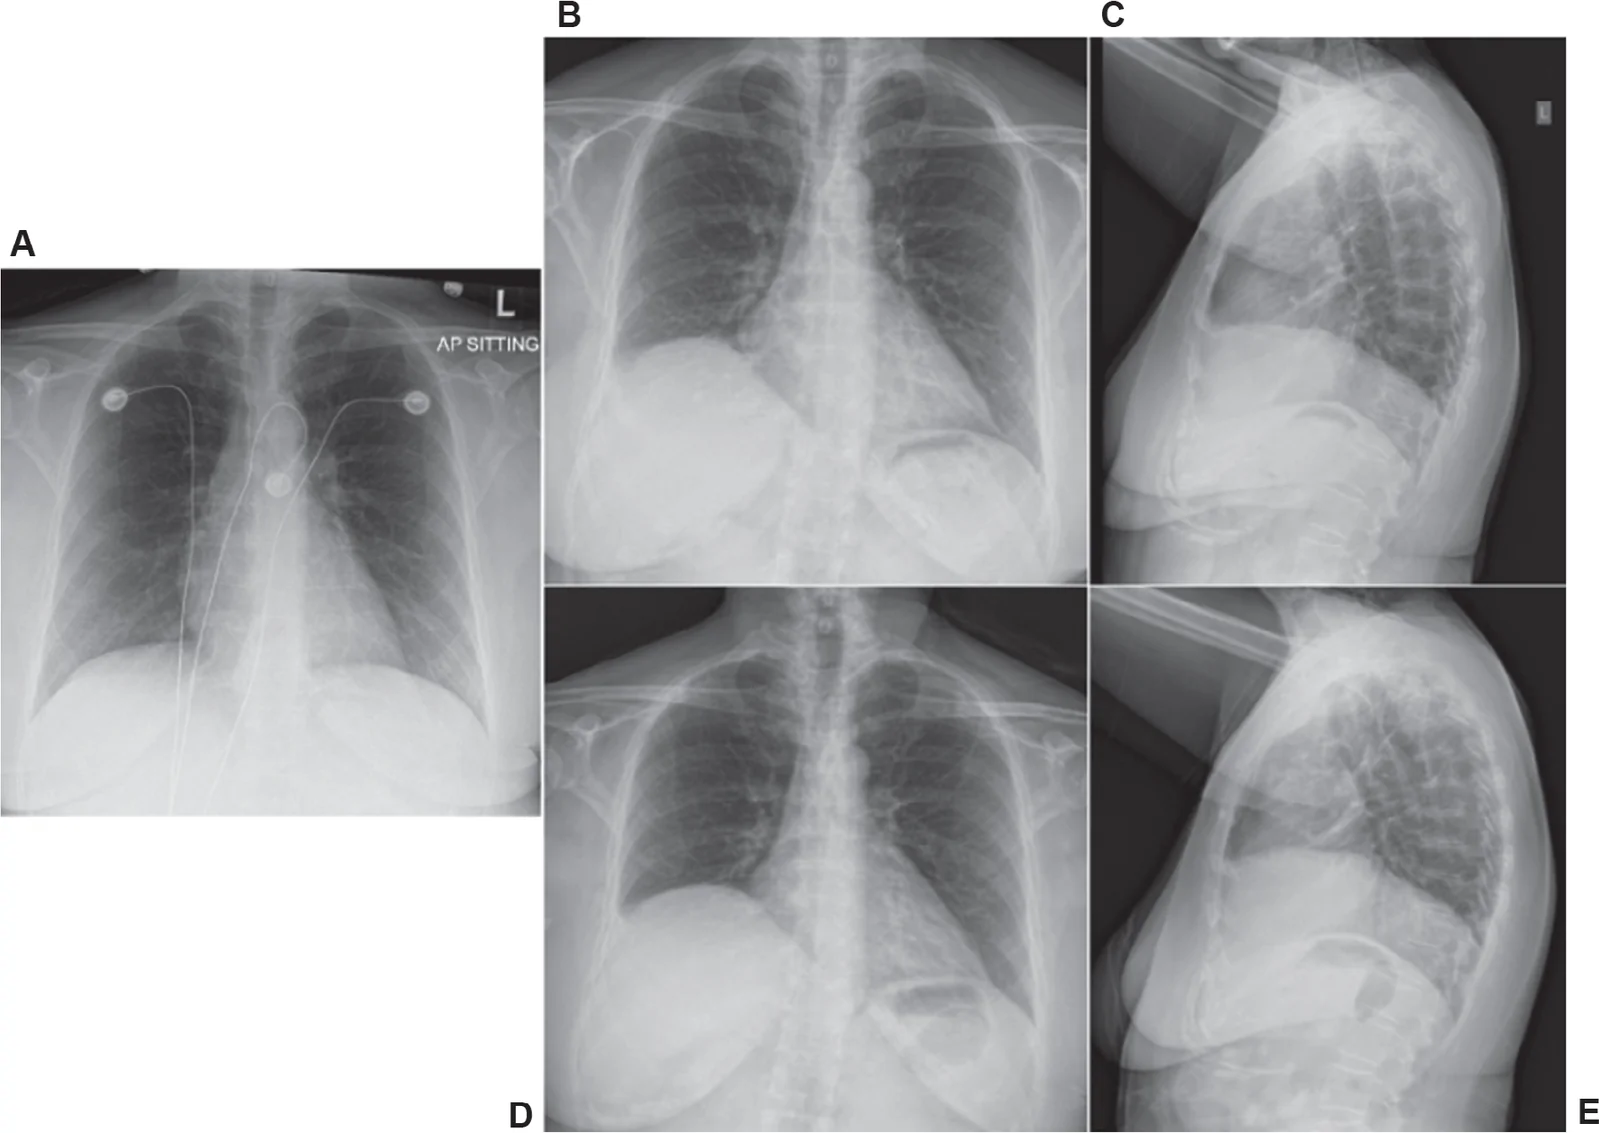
Chest X-ray images. A: Normal antero-posterior chest radiograph pre-ablation. B: Post-procedural antero-posterior chest radiograph showing right hemidiaphragm elevation consistent with diaphragm paralysis. C: Post-procedural lateral chest radiograph showing right hemidiaphragm elevation consistent with diaphragm paralysis. D: Post-procedural 3-month follow-up antero-posterior chest radiograph showing right hemidiaphragm elevation consistent with diaphragm paralysis. E: Post-procedural 3-month follow-up lateral chest radiograph showing right hemidiaphragm elevation consistent with diaphragm paralysis.
